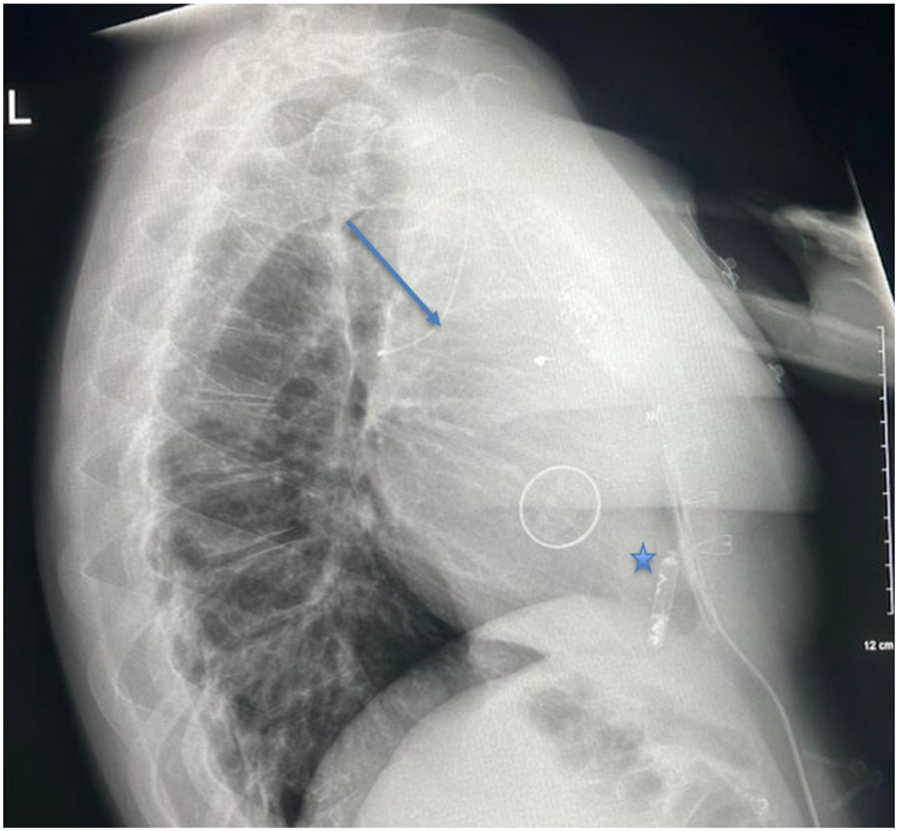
Lateral chest radiograph demonstrating the Abbott AVEIR VR leadless pacemaker in the right ventricular apex and the dislodged SelectSecure Medtronic 3830 lead in the left pulmonary artery branch, confirming post‐procedural stability.
